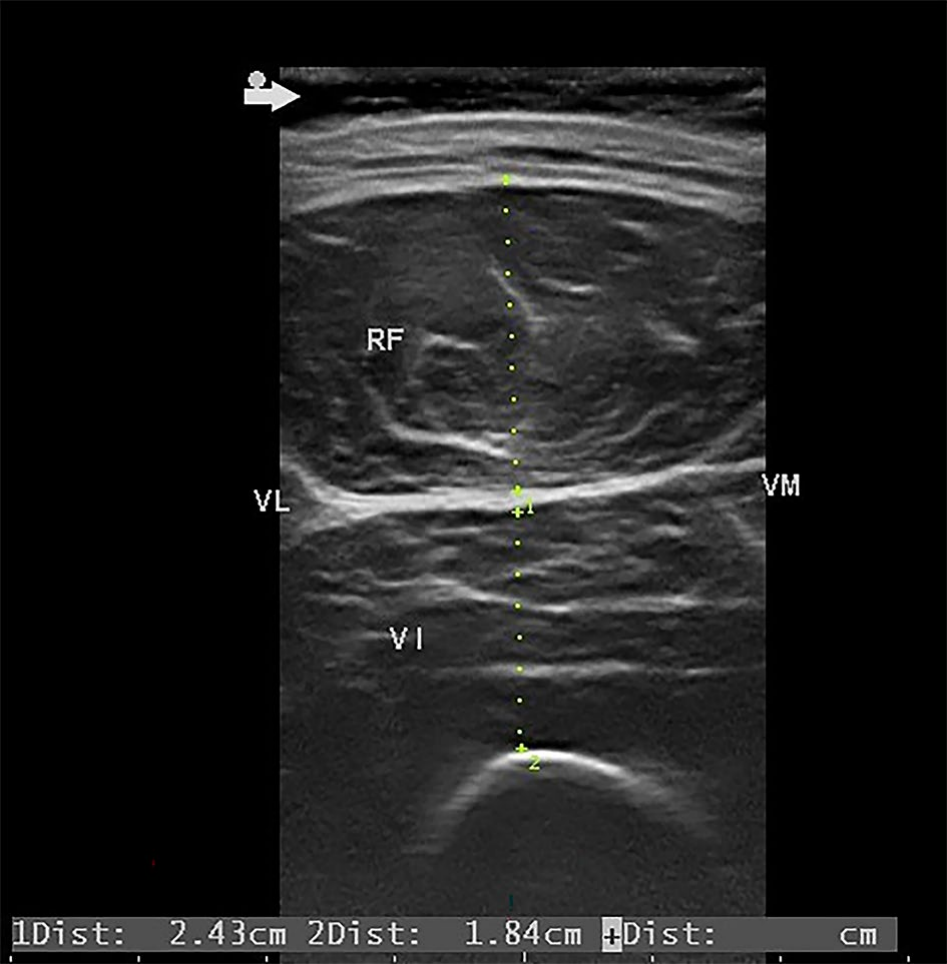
Ultrasound measurements RF: rectus femoris muscle, VI: vastus intermedius muscle, VL: vastus lateralis muscle, VM: vastus medialis muscle, Green dotted line 1: thickness of RF, Green dotted line 2: thickness of VI, Arrow: placement of the gel to ensure that there is no pressure on the muscles to produce deformation.
